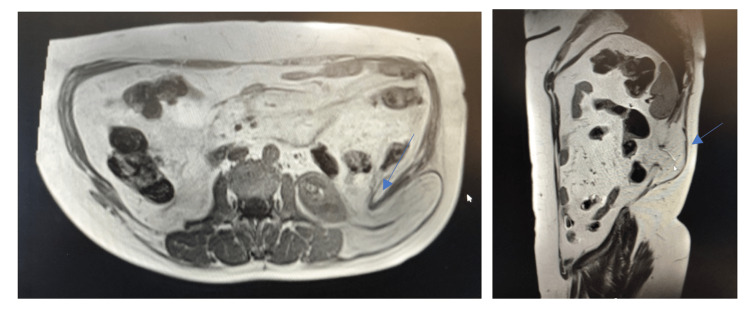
MRI with blue arrows showing herniation through the superior lumbar triangle.
